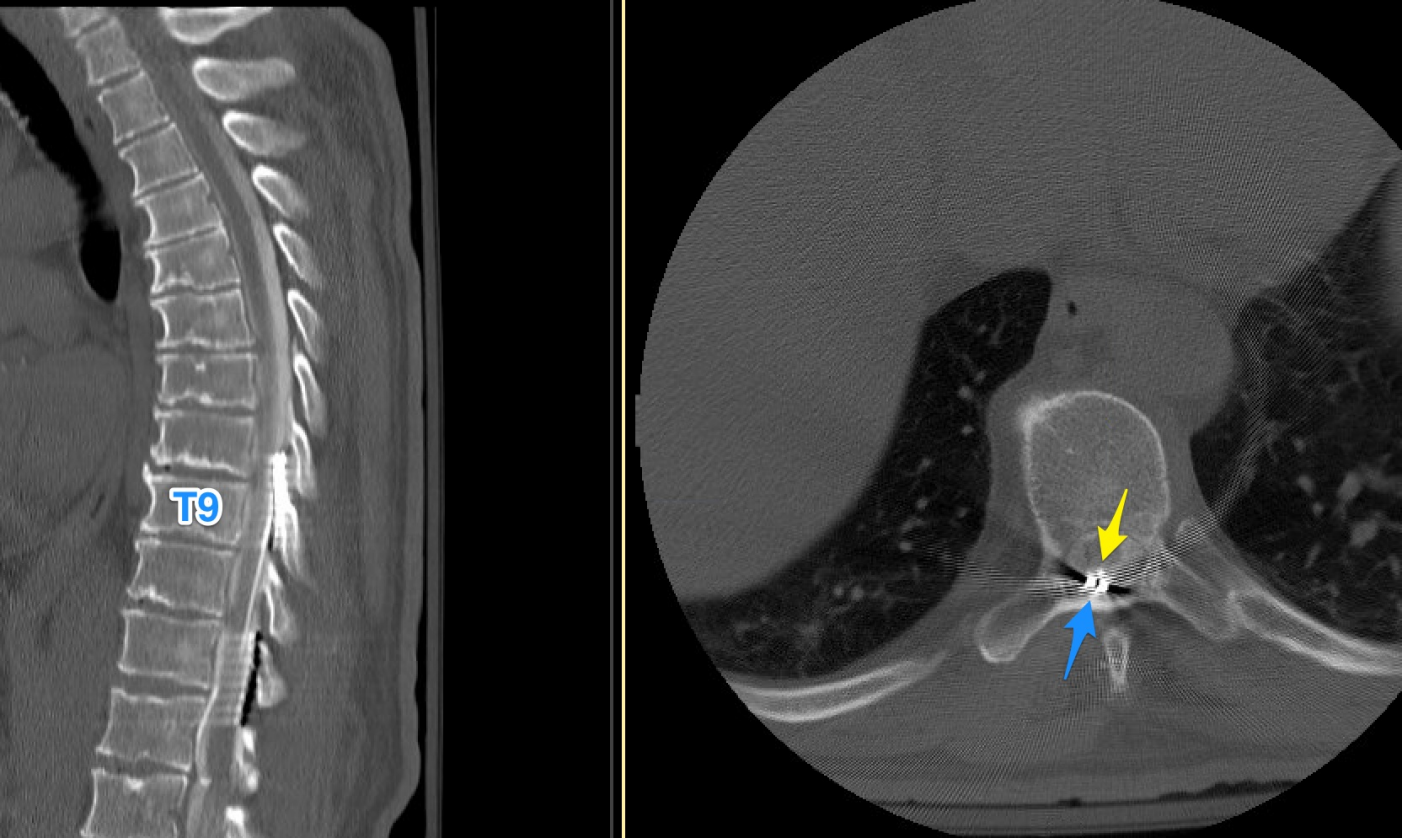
CT myelogram showing the catheter (yellow) and the stimulator lead (blue) tips. No compressive etiology. CT: computed tomography
